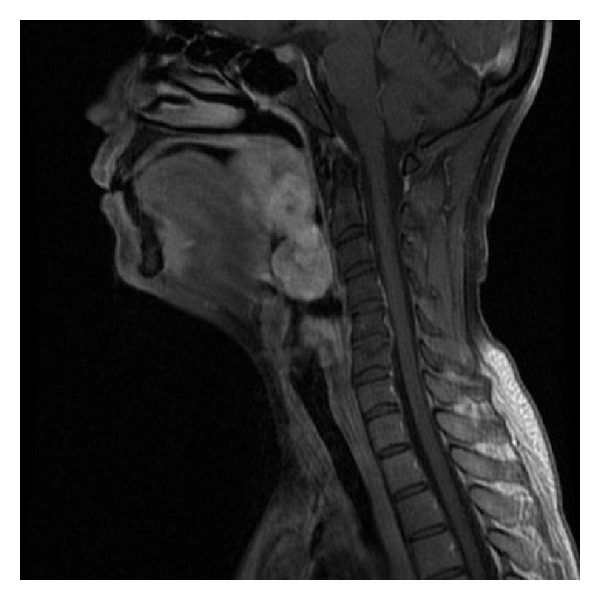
Magnetic resonance imaging of the head and neck assessing the deep extension of the tongue mass.
